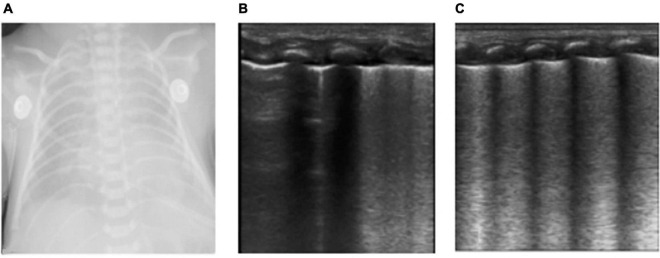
The neonate was born at 36 weeks of gestational age by Cesarean section due to the mother’s central placenta previa hemorrhage. The amniotic fluid was clear, and the Apgar score of 1, 5, and 10 min of birth were 10 points. The neonate had labored breathing and shortness of breath 10 min after delivery and mechanical ventilation was required. It was diagnosed as severe wet lung. (A) Chest X-ray: Lung permeability decreases, and the white lung changes. (B,C) Ultrasound findings: Rough pleural line, reduced A-line, alveolar interstitial syndrome (AIS), double lung points but no consolidation, which conforms to the ultrasonic characteristics of wet lung. After 24 h of treatment, the neonate’s breathing improved. LUS showed that B-lines was significantly reduced and the patient was successfully evacuated from the ventilator. The clinical outcome was consistent with the characteristics of wet lung.
